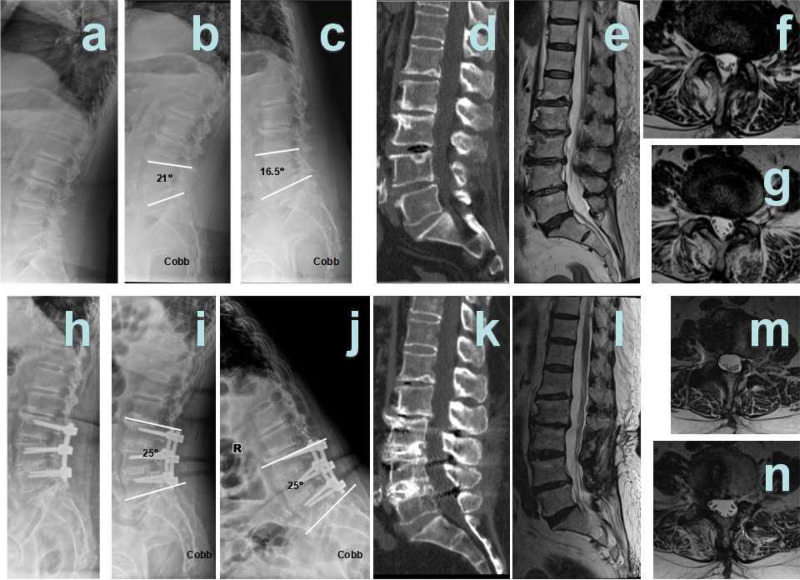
TLIF patient 1. (A) Pre-surgical lateral X-ray; (B) pre-surgical hyper-extension X-ray; (C) pre-surgical hyper-flexion X-ray; (D) pre-surgical CT showed L3/4, L4/5 spinal stenosis; (E) pre-surgical T2 weighted MRI sagittal plane showed L3/4, L4/5 spinal stenosis; (F) pre-surgical MRI showed L3/4 spinal stenosis; (G) pre-surgical MRI showed L4/5 spinal stenosis; (H) 12 months after surgery, post-surgical lateral X-ray; (I) 12 months after surgery, post-surgical hyper-extension X-ray; (J) 12 months after surgery, post-surgical hyper-flexion X-ray; (K) 12 months after surgery, post-surgical CT showed interbody fusion; (L) 12 months after surgery, post-surgical T2-weighted MRI sagittal plane; (M) 12 months after surgery, post-surgical MRI sagittal plane showed that L4/5 spinal stenosis was improved significantly; and (N) 12 months after surgery, post-surgical MRI sagittal plane showed that L5/S1 spinal stenosis was improved significantly.
